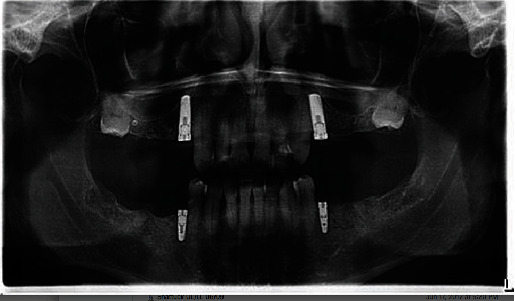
Panoramic radiograph (March 2017) after placement of dental implants and treatment planned for a shortened dental arch.
